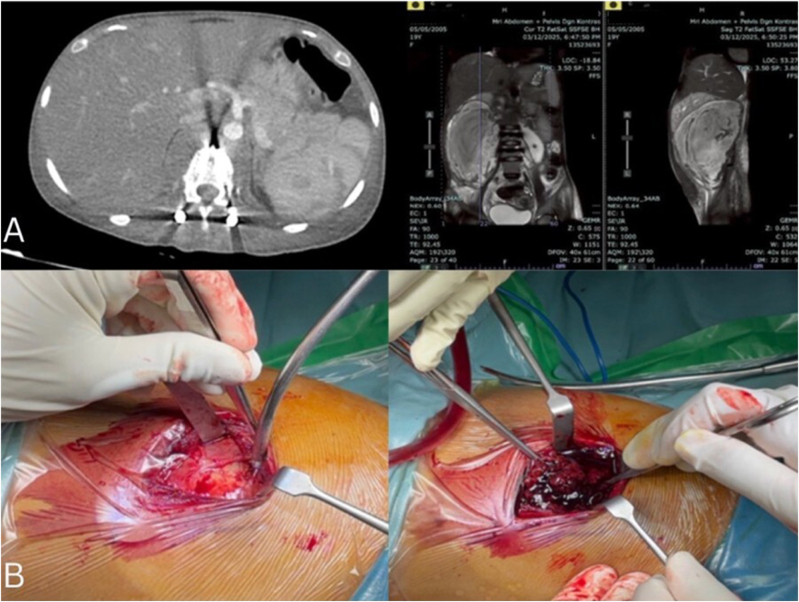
(A) MRI and CT, demonstrated destruction of the L1–L2 vertebral bodies and a large retroperitoneal abscess; (B) First intraoperative picture surgery that shows clot formation in the right abdomen.
